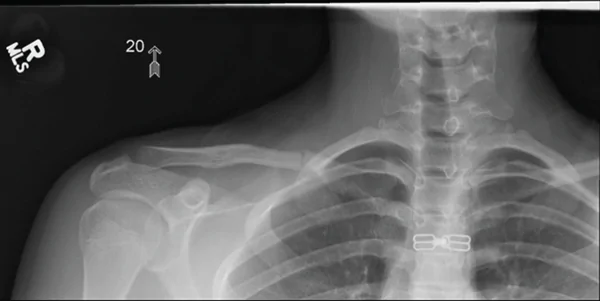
Day 3 radiographs confirming a displaced medial clavicle fracture obtained at initial orthopedic evaluation.
